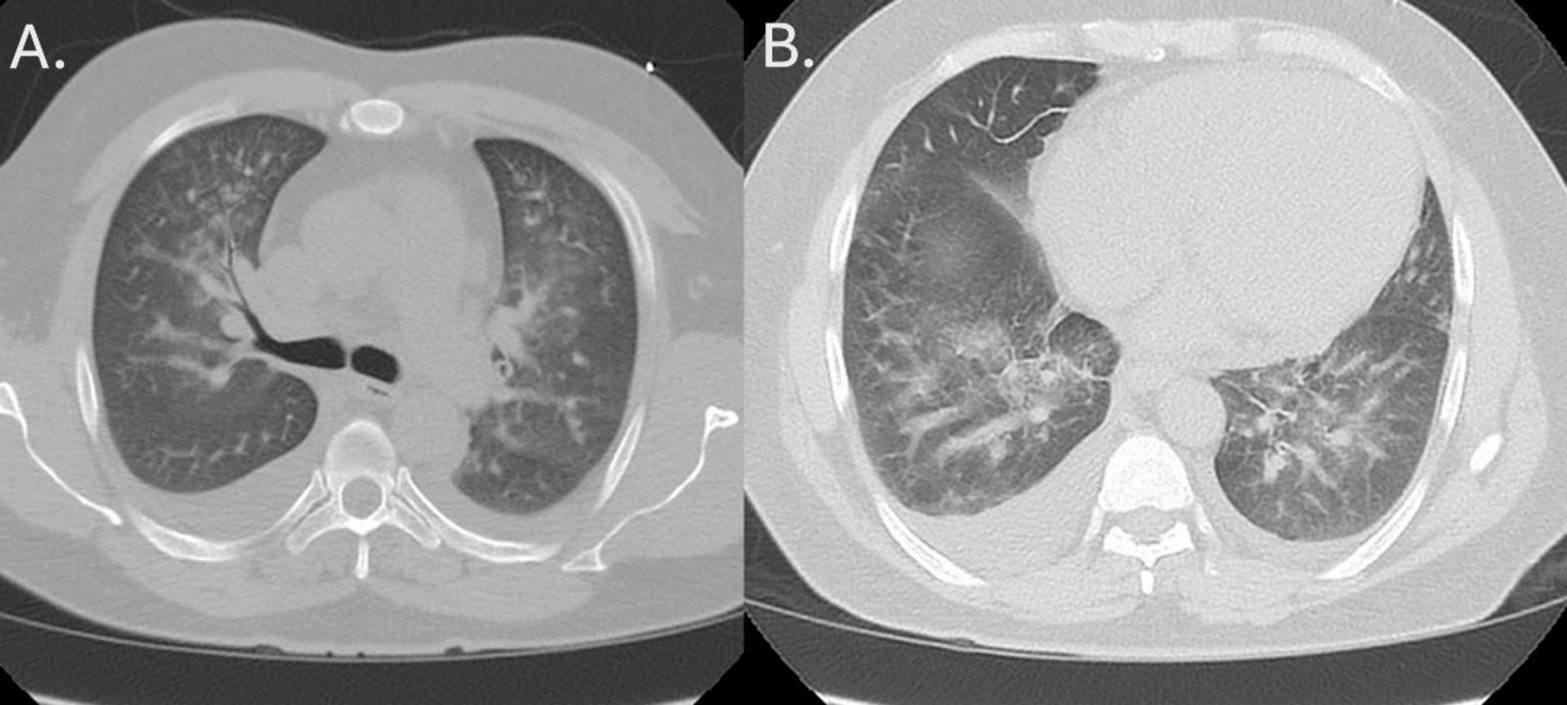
CT of the chest with bilateral ground glass opacities and infiltrates seen at two locations: at the level of the pulmonary arch (A) and below the pulmonary arch (B) CT: computed tomography
